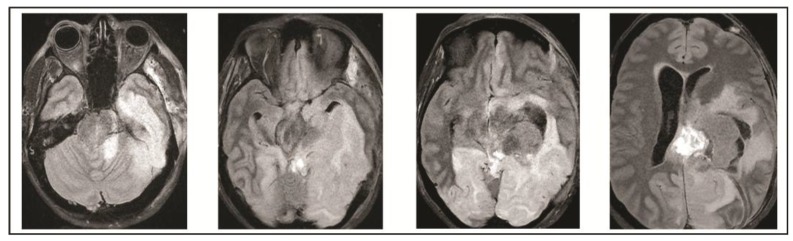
Final MRI on post-operative day 10 obtained demonstrated extensive infarcts secondary to vasogenic edema.
